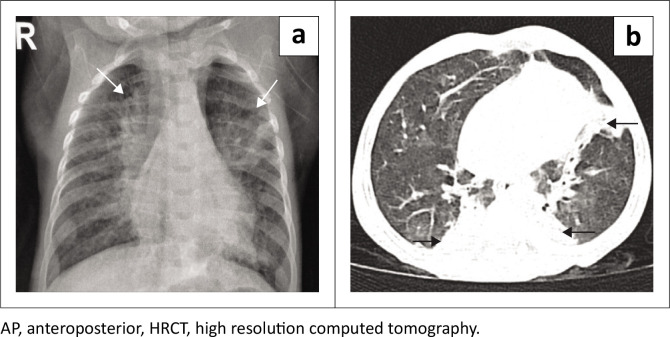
(a) AP chest radiograph demonstrates aspiration changes in the upper zones bilaterally. (b) HRCT thorax of the same patient confirms upper lobe aspiration changes.
